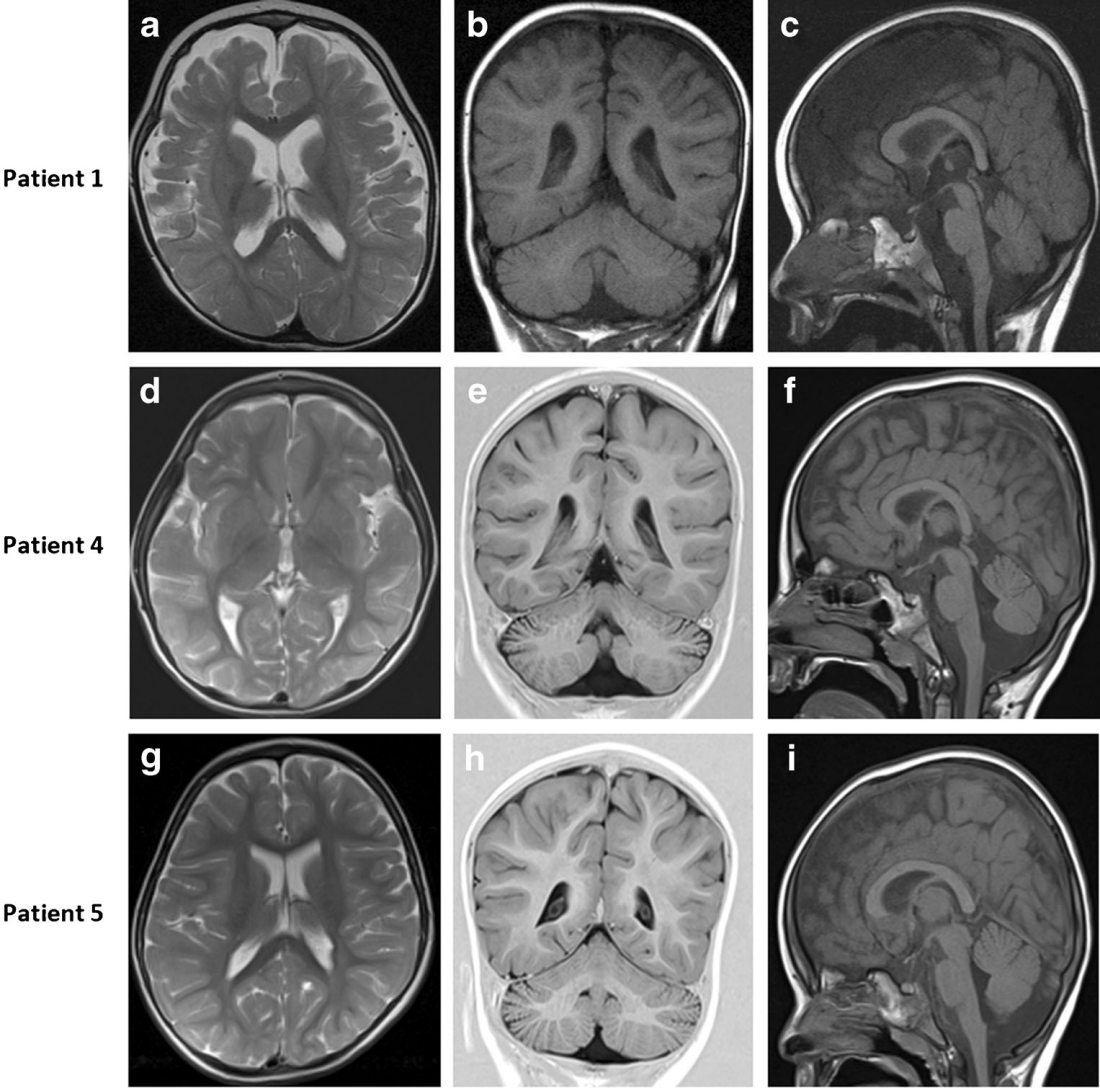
Brain magnetic resonance imaging of patients with SLC25A22 deficiency. (a, d and g) T2-weighted images. (b, c, f and h) T1-weighted images. (e and h) T1-weighted images with inversion recovery sequence. Patient 1 (2 y 1 m), patient 4 (6y 8 m) and patient 5 (2y 10 m). All patients show frontotemporal hypoplasia/atrophy (a, d and g) and prominence of cerebellar folia, consistent with cerebellar hypoplasia/atrophy (b, e and h). All patients also have a small splenium, with the splenium of the corpus callosum smaller than the genu and a small optic chiasma (smaller than the mammillary body) (c, f and i). Abnormal appearance of the insular cortex is also noted in patient 4 (d)
